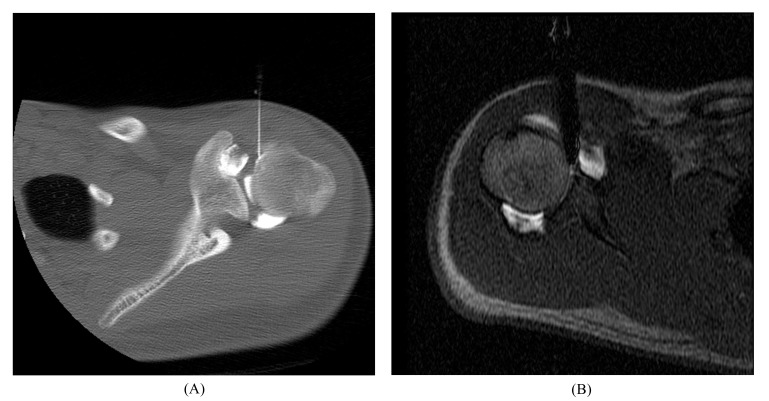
Arthrogram procedure. Anterior approach of needle for image-guided glenohumeral joint injection of contrast material using (A) CT and (B) MRI.
